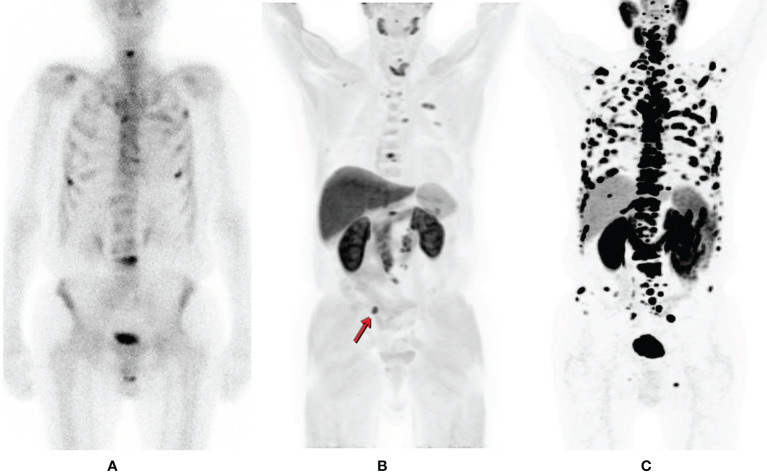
Patient suffering from prostate cancer is referred for bone scintigraphy after biochemical failure, detecting multiple sites of osteoblastic activity corresponding to multiple bone lesions (A). Within one week the patient also underwent a 11C-Choline PET/CT in order to exclude visceral spread (B) for treatment planning. Apart from additional bone secondaries, a common iliac lymph nodal lesion right (arrow) is detected (maximum intensity projection (MIP); (B). However, the true spread of the disease is much more extended by 68Ga-PSMA PET (MIP); (C) [republished with permission of the Hellenic Journal of Nuclear Medicine (7)].
